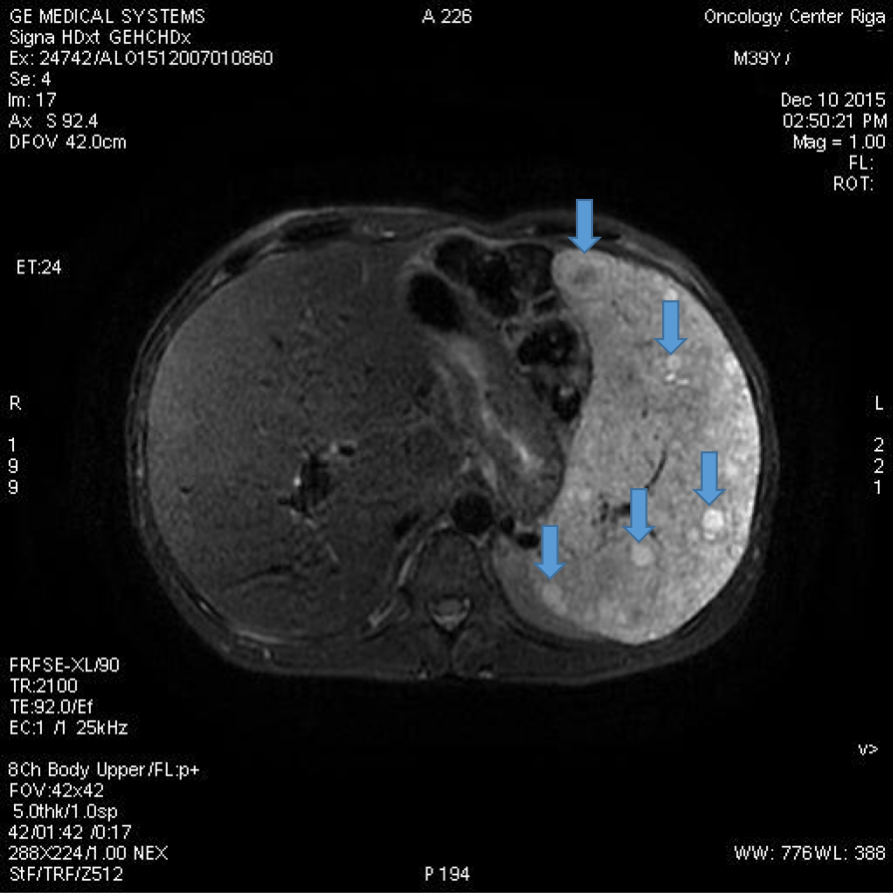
Magnetic resonance imaging (MRI) showing hepatosplenomegaly with multiple hemangiomas in spleen (*arrows*)
